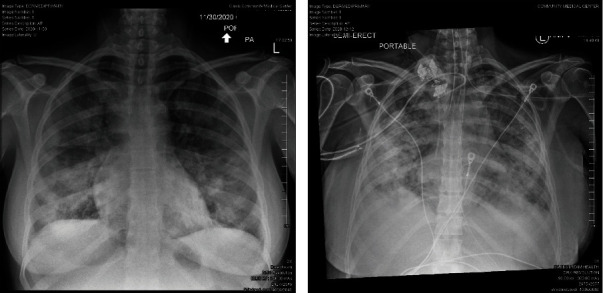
(a) Bilateral infiltrates on initial portable chest X-ray at her first ED visit. (b) Progression of bilateral infiltrates on portable chest X-ray on hospital day 12.
